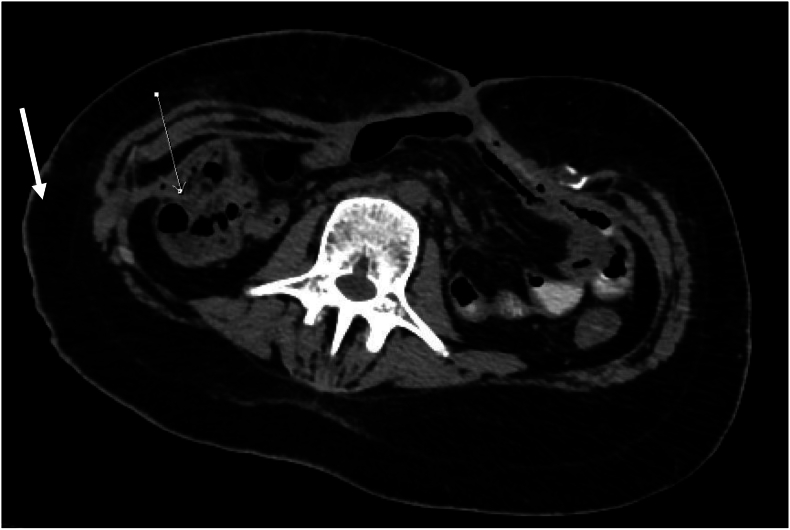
Axial computed tomographic section demonstrating an opacification of the left colon without opacification of the ascending colon, with passage of the contrast medium through the duodenocolic fistula.
